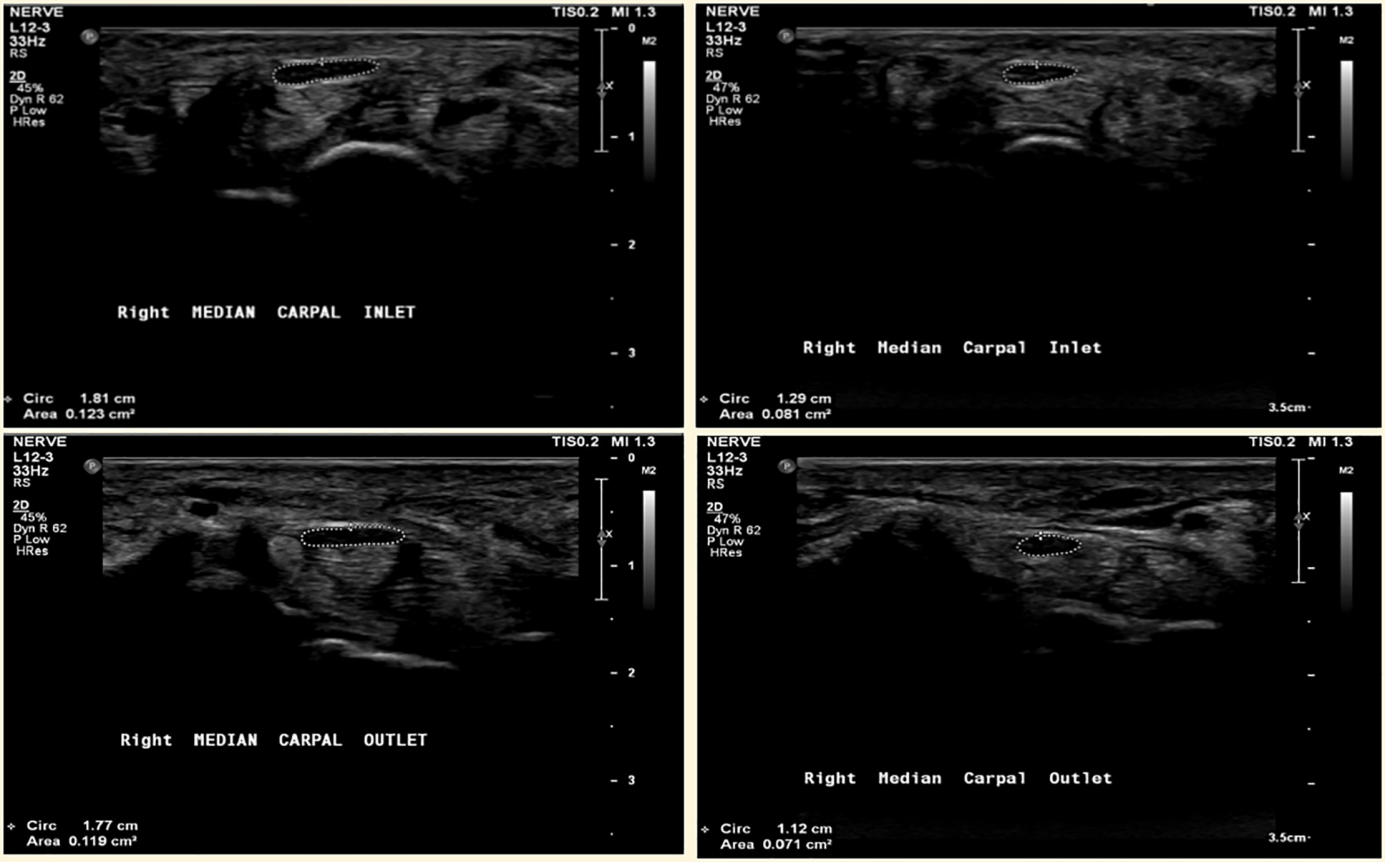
Ultrasound image of the median nerve cross-sectional area (CSA) at the carpal tunnel level. Median CSA of patient (A, B) and healthy control (C, D).
